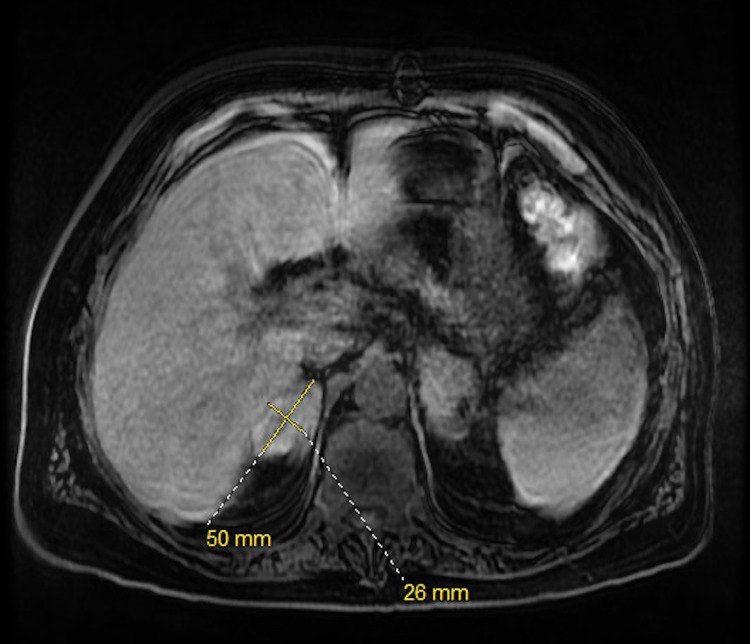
Transversal T1-weighted magnetic resonance image of the abdomen after contrast Transversal T1-weighted magnetic resonance image of the abdomen after contrast showing right adrenal mass measuring 5 cm x 2.6 cm
